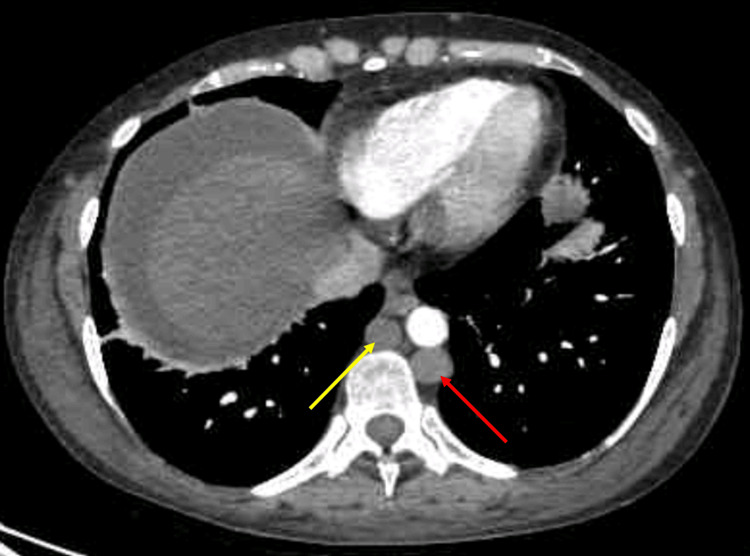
Abdominal/pelvis CTA showing the azygous-hemiazygous system Depicts the azygous-hemiazygous system, with a red arrow denoting the hemiazygous vein and a yellow arrow indicating the azygous vein. These prominent venous systems emerged as a consequence of IVC occlusion secondary to thrombosis. IVC: inferior vena cava; CTA: computed tomographic angiography
